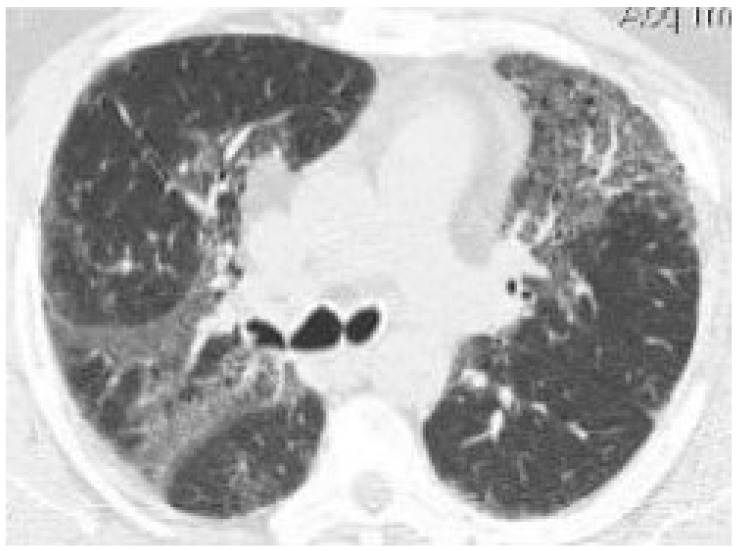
Chest CT of one of the patients with cannabinoid-positive BAL showing bilateral ground-glass opacities (GGO), demarcated by areas of normal lung parenchyma, creating a “mosaic” lung pattern; in the context of the GGO, thickening of the lung interstitium with formation of microcysts can be seen.
